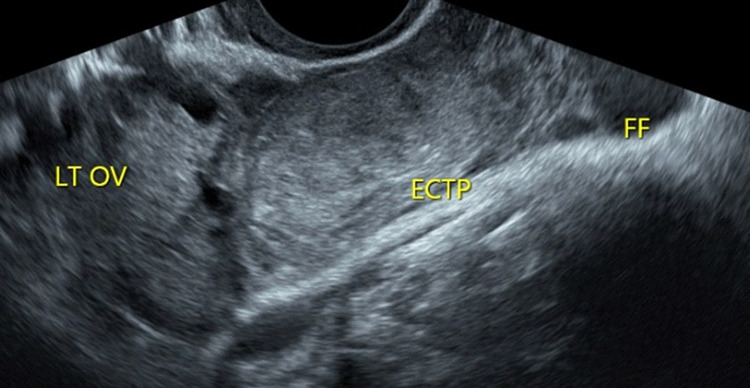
Transvaginal ultrasound scan showing a transverse view of pelvis, with annotation of the left ovary (LT OV), adjacent to the ectopic pregnancy (ECTP) and a small amount of echogenic free fluid (FF) within the adnexa.
